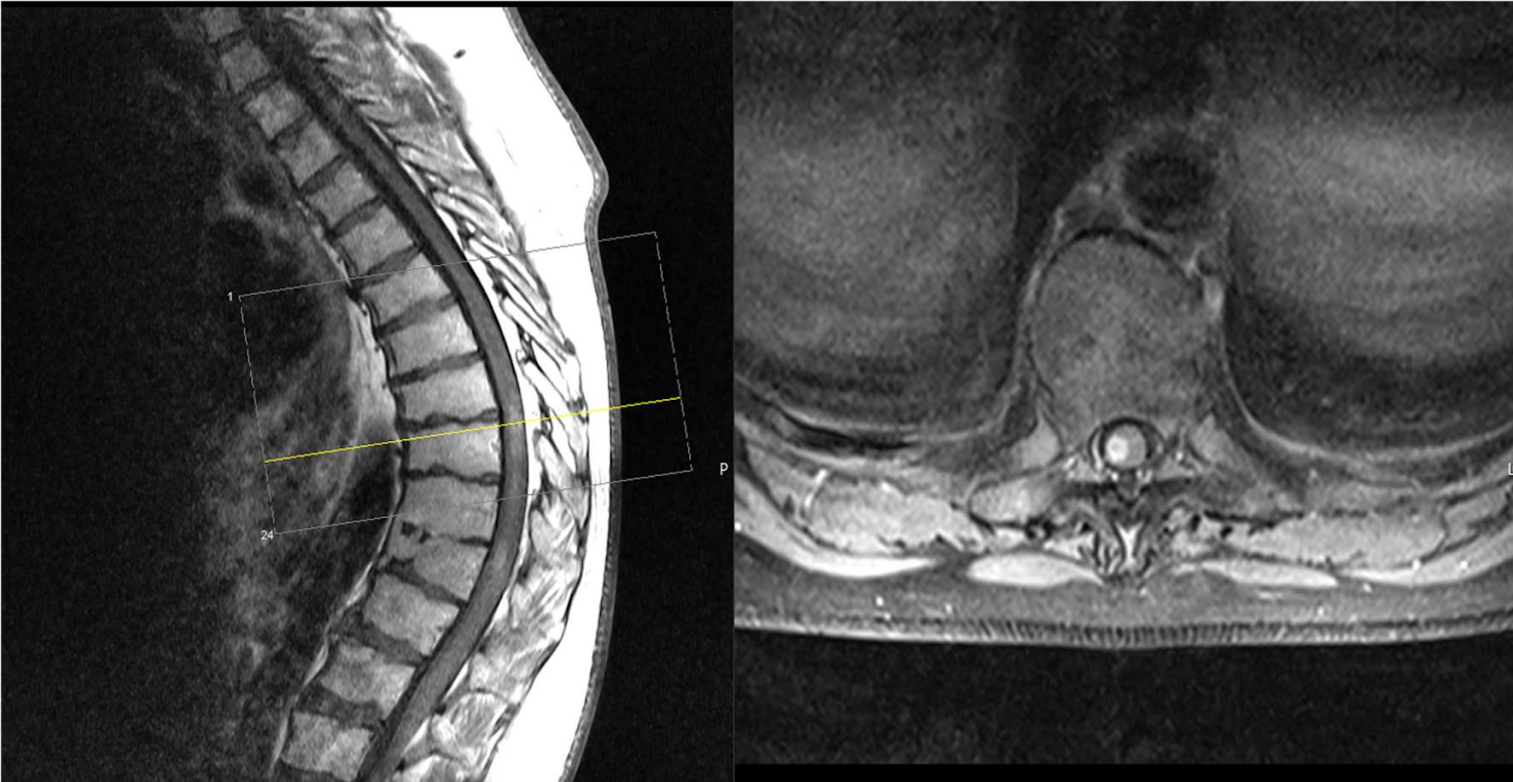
Preoperative MRI (T1 with contrast enhancement) of a patient suffering from an intramedullary Glioblastoma WHO IV at the level Th8-10
